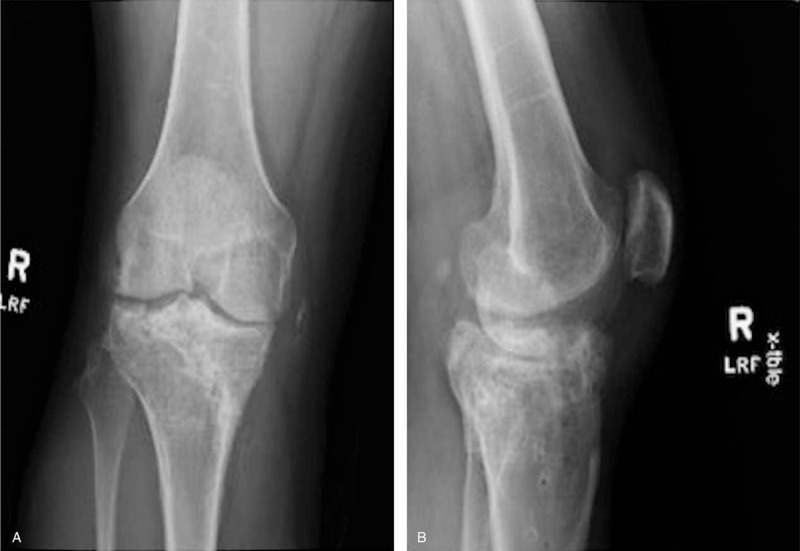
2A: AP preoperative x-ray demonstrating hardware removal, and end-stage posttraumatic arthritis. 2B: Lateral x-ray of Figure 2A.
